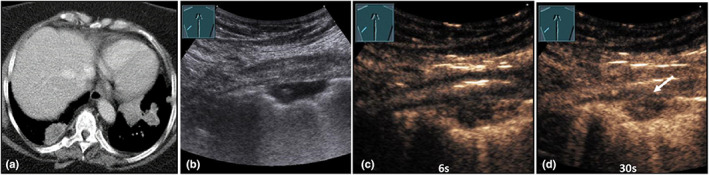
A 56‐year‐old female patient presented with fever, dyspnea, ear pain, weight loss, and shortness of breath without improvement after antibiotic therapy; there was laboratory evidence of positive perinuclear anti‐neutrophil cytoplasmic antibody levels on serologic examination. Computed tomography (courtesy of Prof. Dr. Andreas H. Mahnken, Department of Radiology, University Hospital Marburg) (a) shows multiple predominantly right‐sided consolidations; B‐mode ultrasound (b) shows a left‐sided, hypoechoic, pulmonary limited consolidation; contrast‐enhanced ultrasound shows reduced bronchial arterial perfusion after 6 s (c) and after 30 s (d) with areas of absent perfusion (arrow). Ultrasound‐guided biopsy revealed the diagnosis of granulomatosis with polyangiitis.
